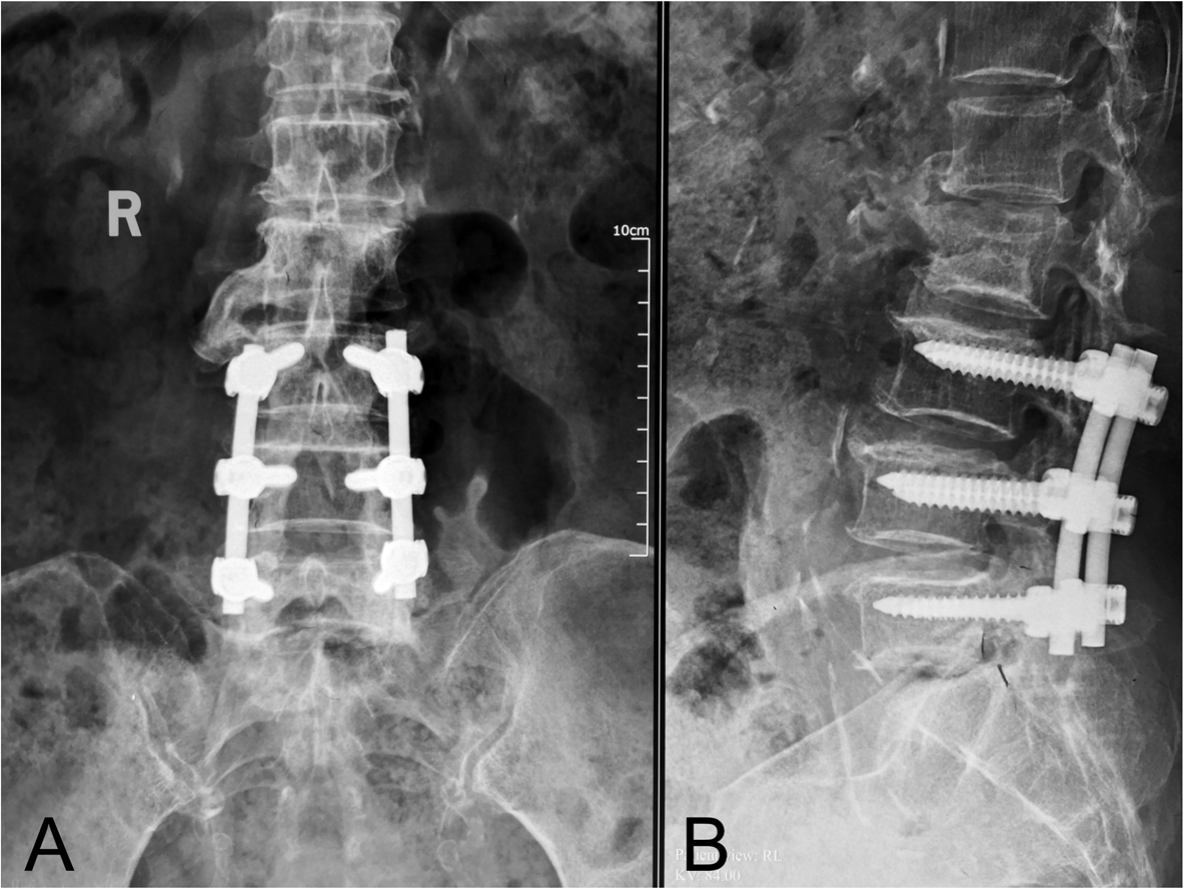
Anterior-posterior (AP) and lateral X-ray images of the lumbar spine demonstrated a vertebral compression fracture of L5 with good alignment of the construct from L3–5
